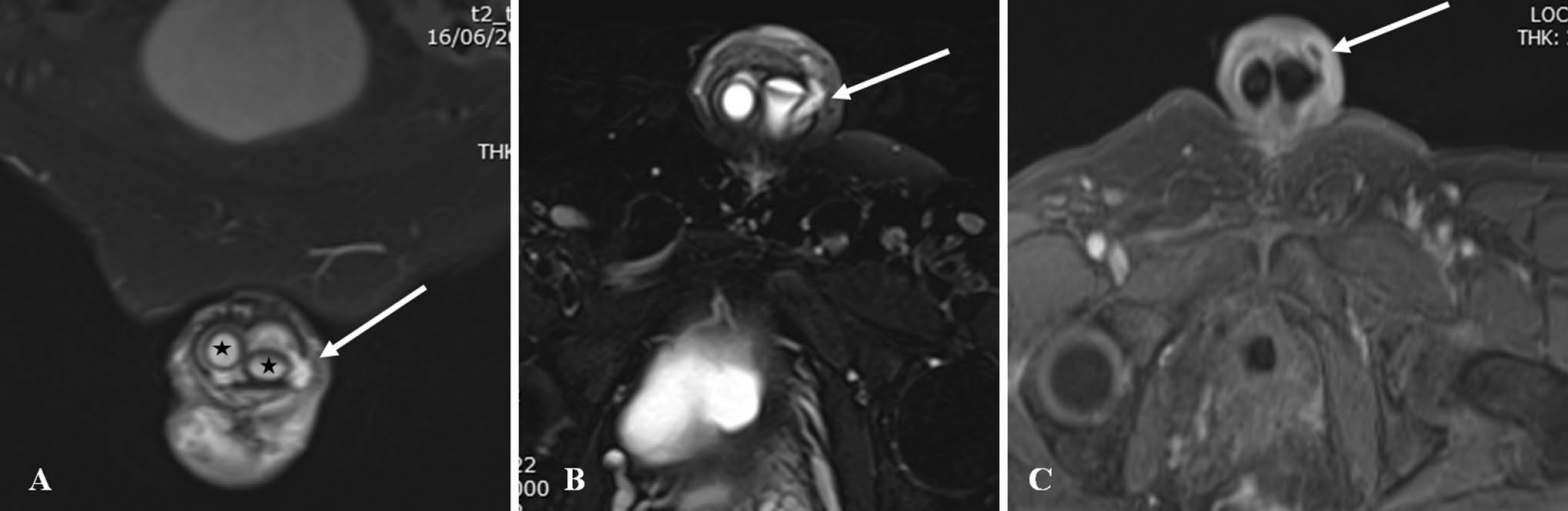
59-year-old diabetic man presented with wound discharge close to tubing site 25 days after penile prosthesis placement. Coronal (a), axial (b) T2-weighted and axial post-contrast (c) MRI images show small fluid collection with peripheral enhancement extending through the tunical defect into subcutaneous plane (arrow). Two corporal cylinders are seen (asterisk)
